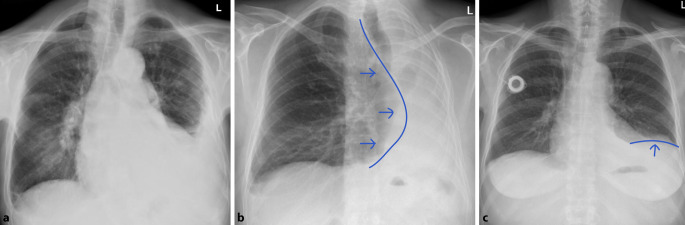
Schwieriger ist es bei der Differenzialdiagnose *Pleuraempyem*. Das typische Muster ist eine linsenförmige Verdickung der Pleura mit Aussparung des Recessus. Jedoch ist eine Beurteilung auch mit einer ergänzenden CT-Untersuchung oft nicht konklusiv möglich. Zusätzliche Zeichen wie eine pleurale Verdickung oder eine vermehrte Kontrastmittelanreicherung treten oft erst spät auf [17].
